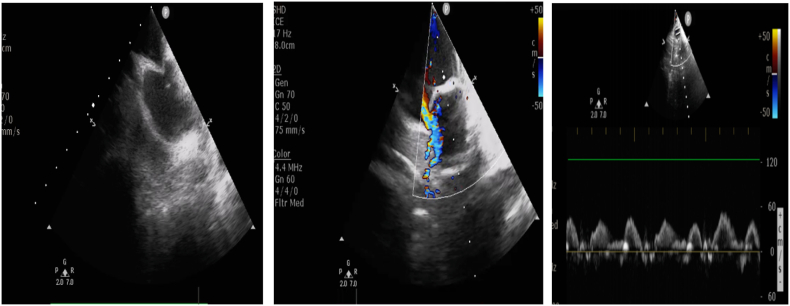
The utility of ICE in cryoablation. (*Left),* tenting of the interatrial septum by the septal puncture needle during LA entry (video 3), (*middle)*, image assessing for leak during balloon occlusion and monitoring of phrenic nerve function using pulsed wave Doppler using ICE (right).
